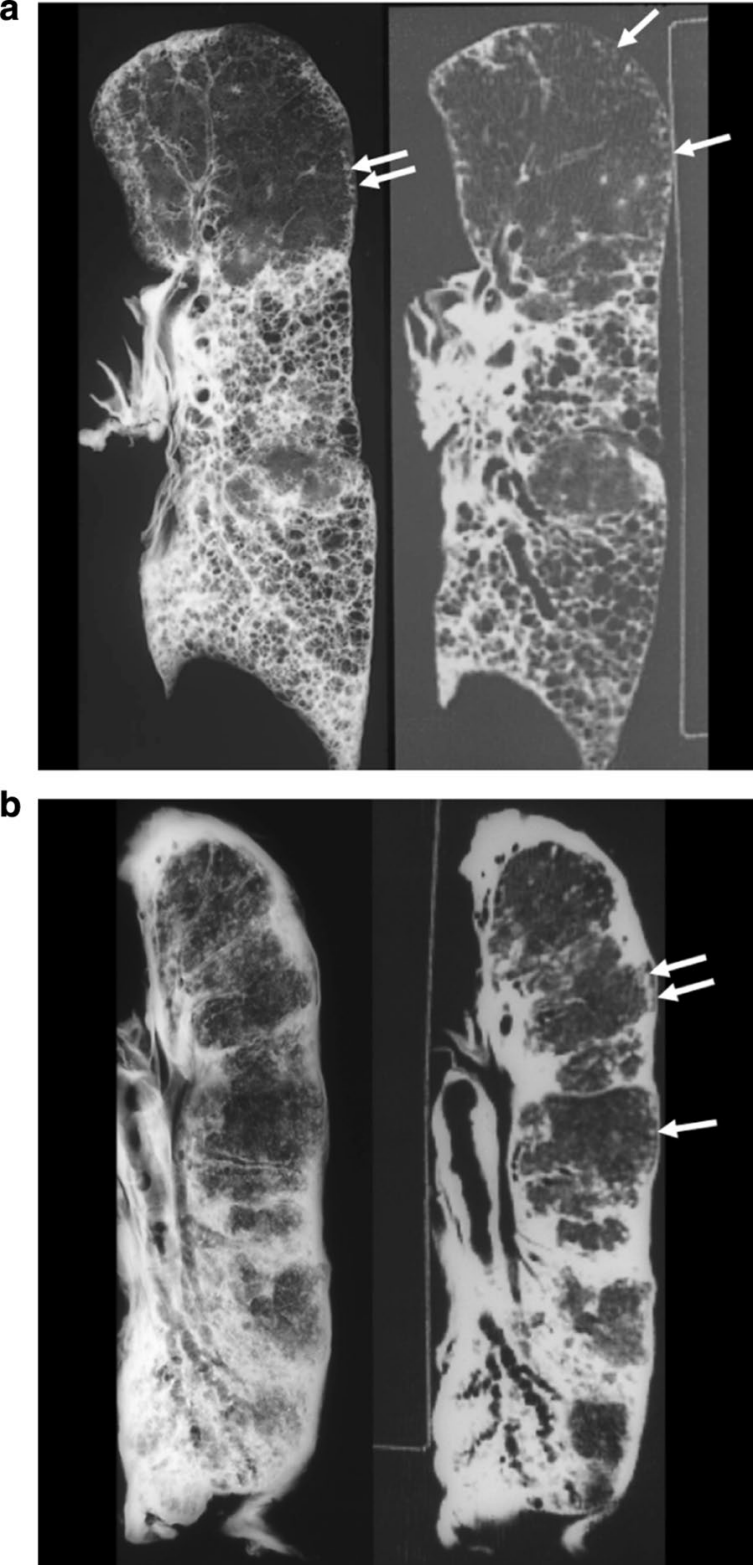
Two types of advanced asbestosis. a A honeycomb type of asbestosis. Postmortem low-kilovoltage radiograph and HRCT scan of the inflated and fixed left lung shows honeycombing in the lower two-thirds of the lung. In the subpleural zones of upper lung, dot-like lesions are seen (arrows). b An atelectatic fibrosis type of asbestosis. Postmortem low-kilovoltage radiograph and HRCT scan of the inflated and fixed left lung shows diffuse pleural thickening and consolidated area extending along the bronchovascular sheath. Traction bronchiectasis is seen in the consolidated area. Honeycombing is not seen radiologically. In the subpleural zones of upper lung, subpleural dots and subpleural lines are also seen (arrows)
